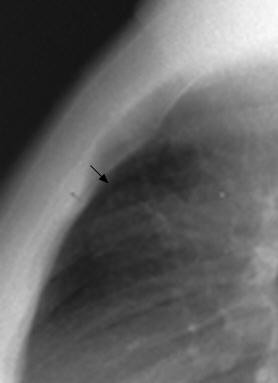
A profile chest x-ray showing a retrosternal tumor.
